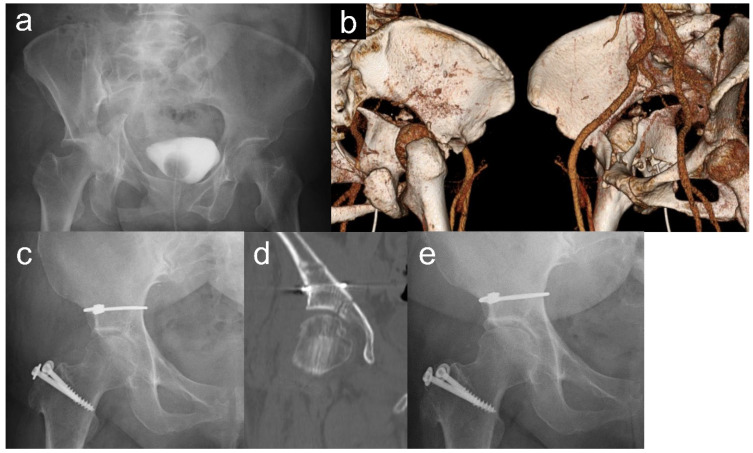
A case presentation of a 60-year-old woman injured due to a traffic accident. (a,b) Preoperative plain radiograph and computed tomography (CT) showed a T-shaped acetabular fracture. (c,d) Postoperative plain radiograph of cerclage cable fixation. The reduction quality is seen to be anatomical. (e) Plain radiograph at the 13-year follow-up. No osteoarthritis and no heterotopic ossification can be observed.
